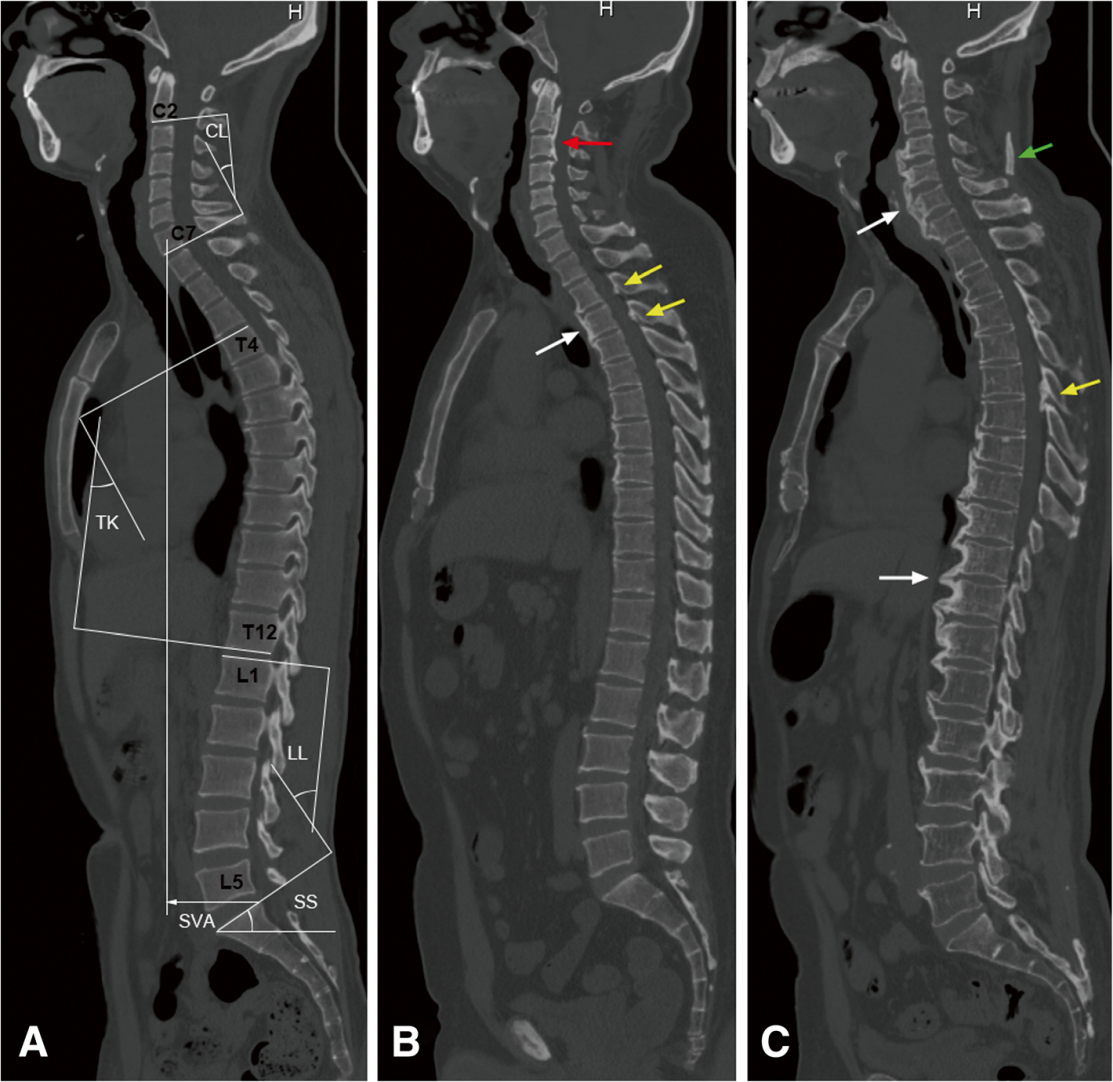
Sagittal radiologic parameters and examples of each type of OSL on whole spine computed tomography. a Measurement methods for CL, TK, LL, SS, and SVA. b A 50-year-old man had OPLL (red arrow), OLF (yellow arrow), and OALL (white arrow). c A 70-year-old man had OLF (yellow arrow), DISH (white arrow), and ONL (green arrow). Abbreviations: CL, cervical lordosis; TK, thoracic kyphosis; LL, lumbar lordosis; SS, sacral slope; SVA, sagittal vertical axis; OPLL, ossification of the posterior longitudinal ligament; OLF, ossification of the ligamentum flavum; OALL, ossification of the anterior longitudinal ligament; DISH, diffuse idiopathic skeletal hyperostosis; ONL, ossification of the nuchal ligament
